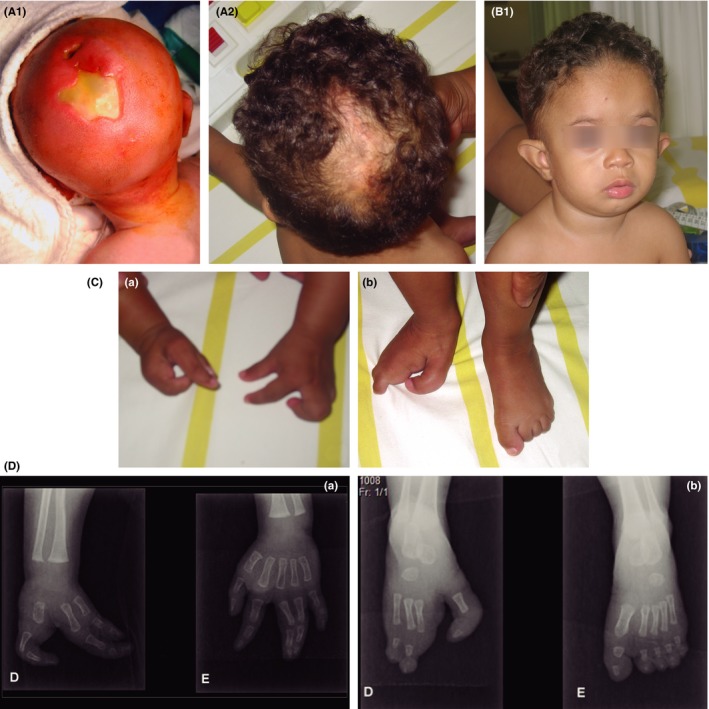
(A1) Patient at one day after birth, showing cutis aplasia in midline scalp before surgical correction; (A2) Cutis in midline scalp at one year and 20 days‐old after the correction of the cutis aplasia; (B1) Patient at one year and 20 days‐old, note high forehead, micrognathia, low set, and poorly folded ears; (C(a)) Note ectrodactyly on the hands; (C(b)) Ectrodactyly on the feet; (D(a)) Radiograph of right hand shows a typical cleft hand deformity with absence of the central rays (the third and fourth rays); radiograph of left hand shows a complete simple syndactyly of third and fourth rays. (D(b)) Radiograph of right foot shows a typical cleft feet deformity with complete absence of second ray, third metacarpal was present but phalanges of middle finger were absent; complete simple syndactyly of 4th and 5th rays, and lateral deviation of the hallux. Radiograph of left foot shows a partial simple syndactyly from second to fifth toes
